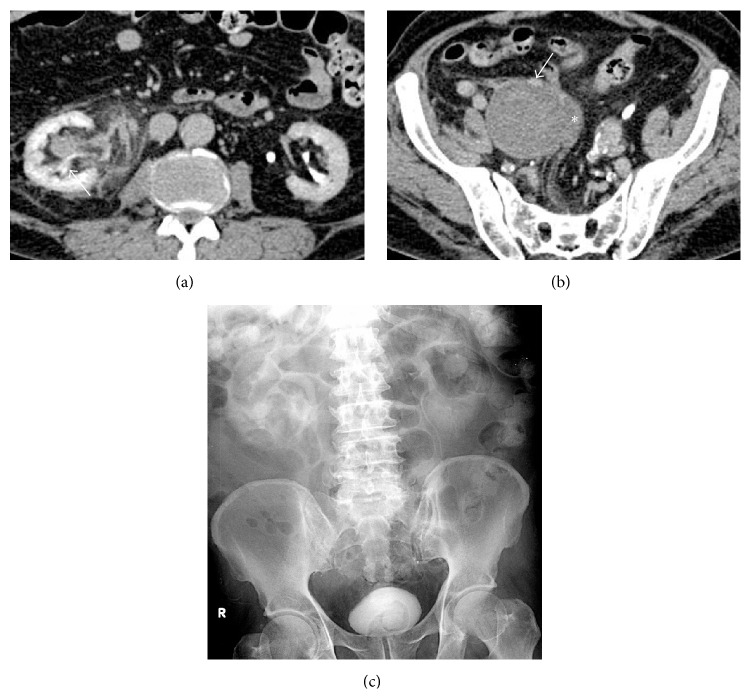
(a) The abdominal CT scan demonstrates fornix rupture with urine extravasation (arrow) around the right kidney and ureter. (b) A saccular right internal iliac artery aneurysm with thick mural thrombus compressing the right midureter (arrow) was noted. (c) Plain abdomen after CT scan shows right perinephric contrast media collection.
